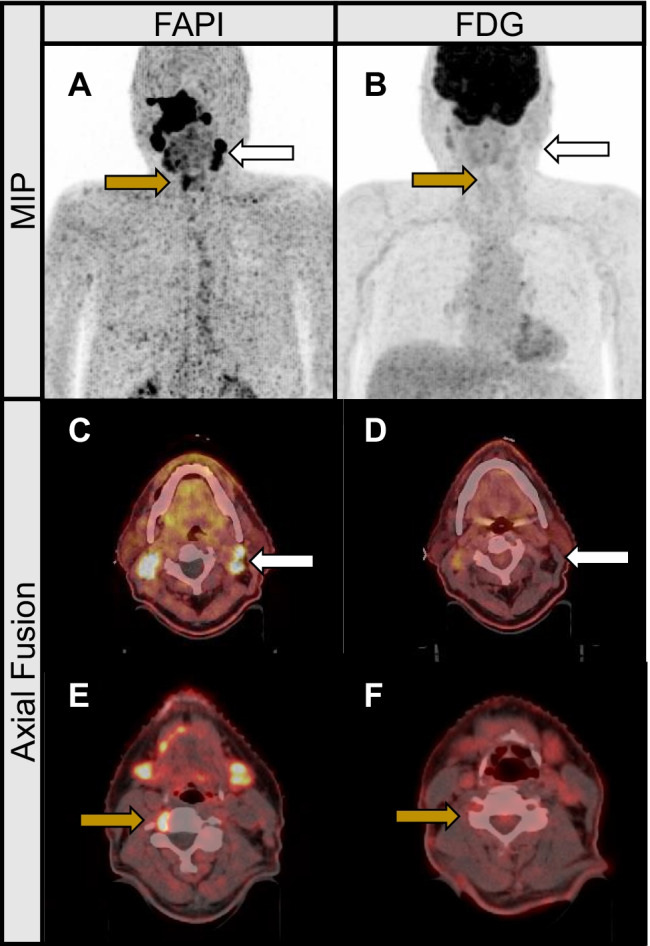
Cervical nodal metastasis with high FAPI uptake and low FDG uptake maximum intensity projections (A, B) and axial fusion images of a 73-year-old male with nasopharyngeal cancer prior to radiation therapy. The patient was upstaged with newly emerged nodal metastasis on both sides of the neck, noticed only in the FAP scans (C, D; white arrows). [.^68^ Ga]-FAPI PET/CT also revealed one cervical lymph node metastasis in close proximity to the cervical spinal cord (at C4 level) that would have been missed in the FDG-scans (E, F; yellow arrows).
